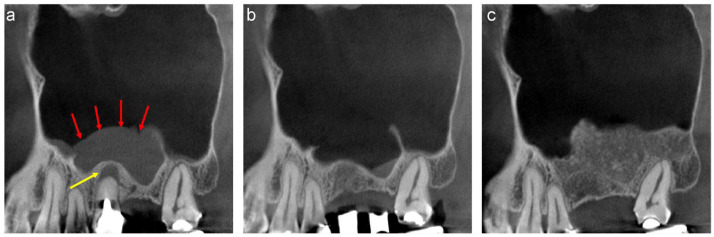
The mesiodistal section of CT images of the left maxillary sinus. (a) Well-defined, faintly radiopaque antral lesions (red arrows) and bone resorption at the palatal root apex of 26 (yellow arrow) are observed. (b) Almost all well-defined, faintly radiopaque antral lesions had disappeared four months after the extraction. (c) A radiopaque area is observed at the sinus floor elevation without swelling of the sinus membrane nine months postoperatively.
